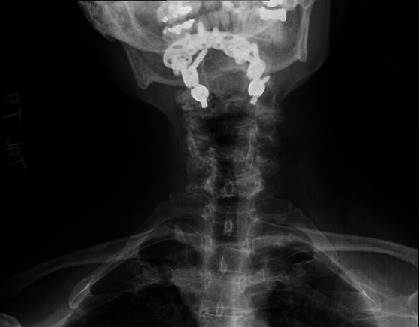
Postoperative AP cervical X-ray showing improvement in lateral deviation of the head following fusion.
